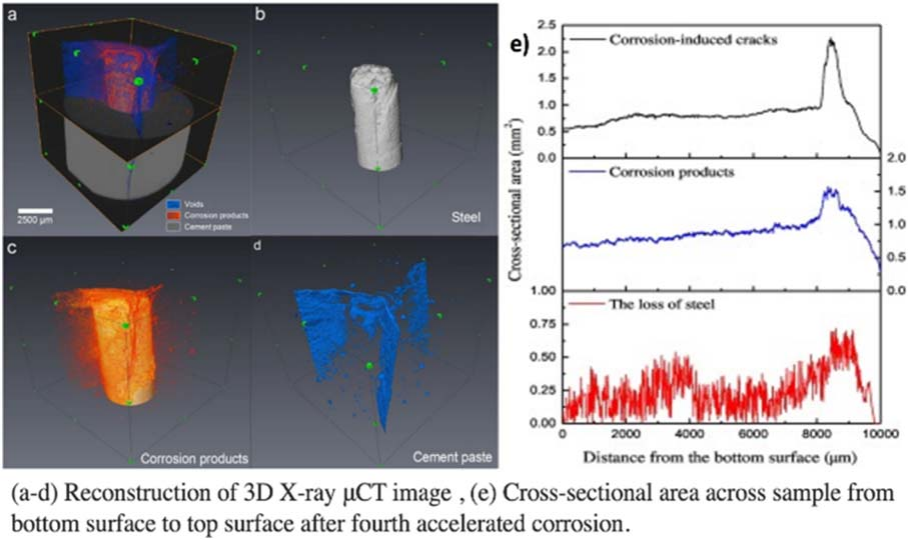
X-ray micro computed tomography results.^42^
